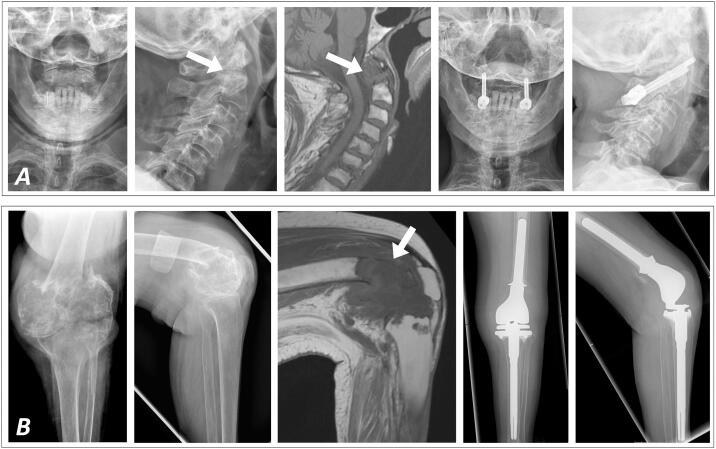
Both the dens (A; pre- and postoperative X-rays anteroposterior and lateral as well as MRI T1 sagittal) and the left distal femur diaphysis (B; pre- and postoperative X-rays anteroposterior and lateral as well as MRI T1 sagittal) sustained pathological fractures requiring operative treatment. As osseous destruction at both sites was advanced, osteosynthesis was not feasible anymore but fusion of C1 and C2 was necessary at the cervical spine while distal femur resection and reconstruction by tumor endoprosthetic replacement was performed at the left femur and knee joint, followed by radiation therapy. The postoperative course at both locations was uneventful, the patient was mobilized without restrictions and free of complaints after the rehabilitation.
